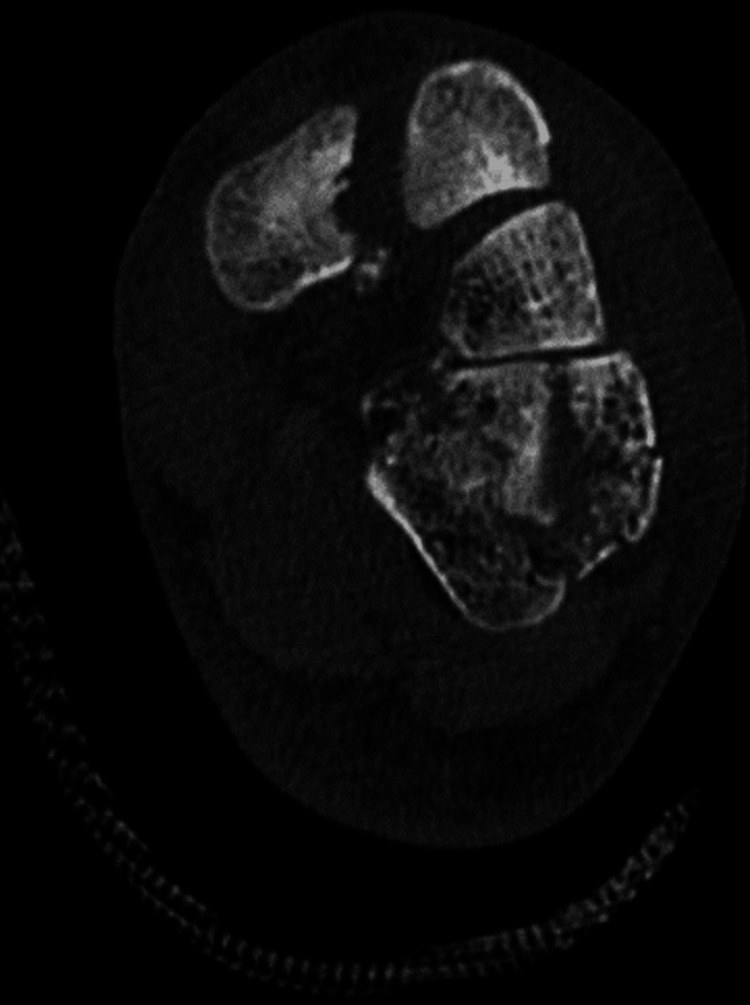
CT scan showing cuboid and cuneiform fractures
